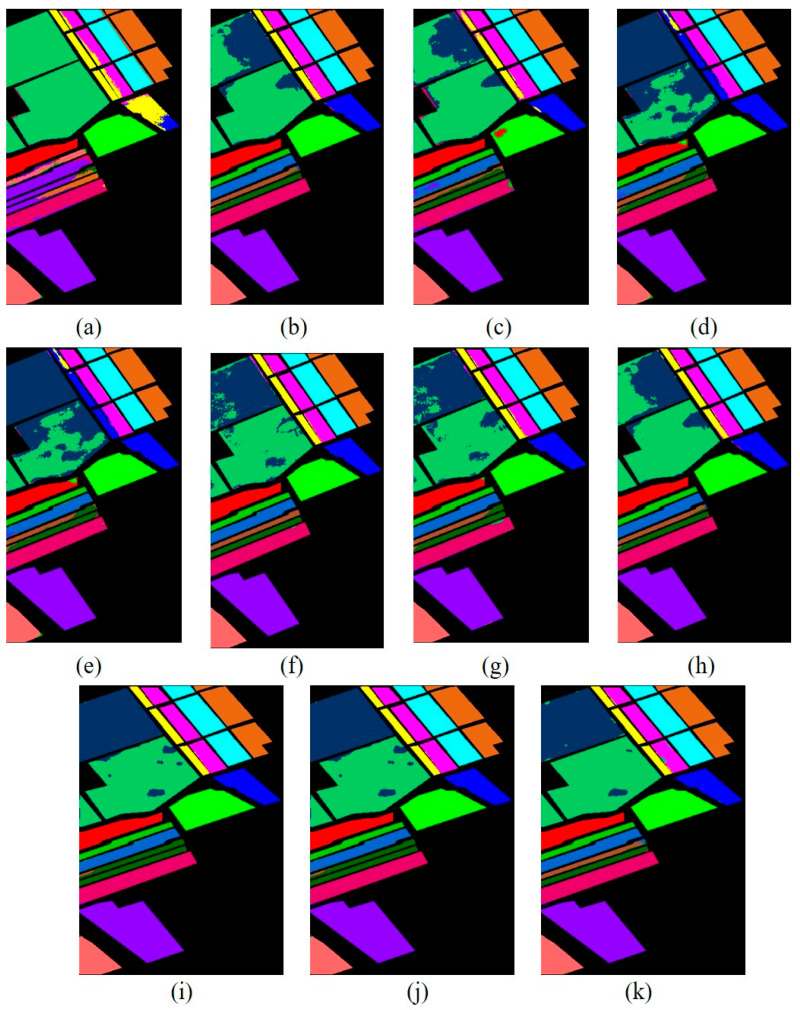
Classification maps of different methods for Salinas dataset. (a) SVM, (b) 2D, (c) 3D, (d) Hybrid, (e) DFFN, (f) Bam-CM, (g) ViT, (h) ST, (i) CT Mixer, (j) SSFTT, and (k) proposed.
